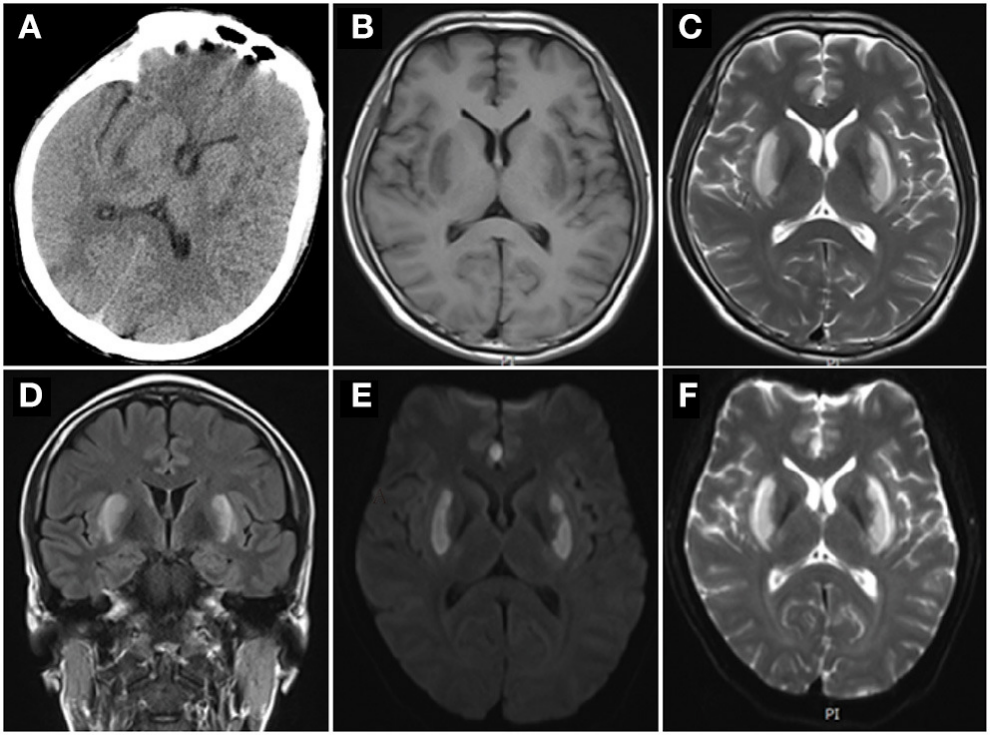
Brain images. (A) CT scan showed bilateral symmetric basal ganglia low density. Brain MRIs showed low signal intensities in the bilateral symmetric putamen in T1 (B), and high signal intensities in T2 (C), Axial FLAIR (D), diffusion weighted image (E), apparent diffusion coefficient (F) over the same regions.
